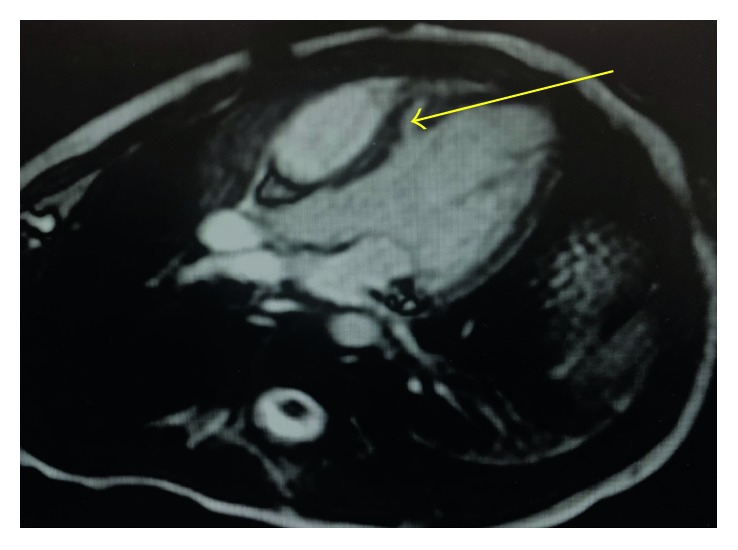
Cardiac MRI (multislice 4-chamber view) showing a small muscular VSD (yellow arrow).
